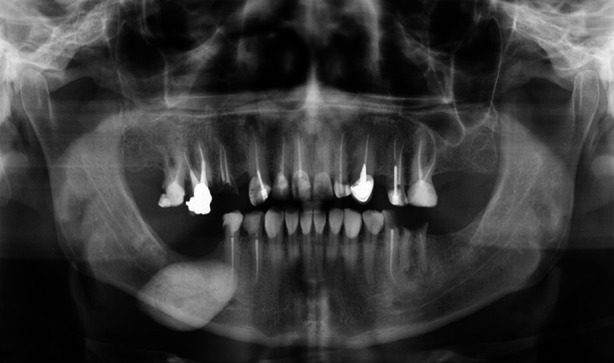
The Panoramic radiograph shows a single, roughly oval, large, well-defined radiopaque mass in the right body of the mandible.
